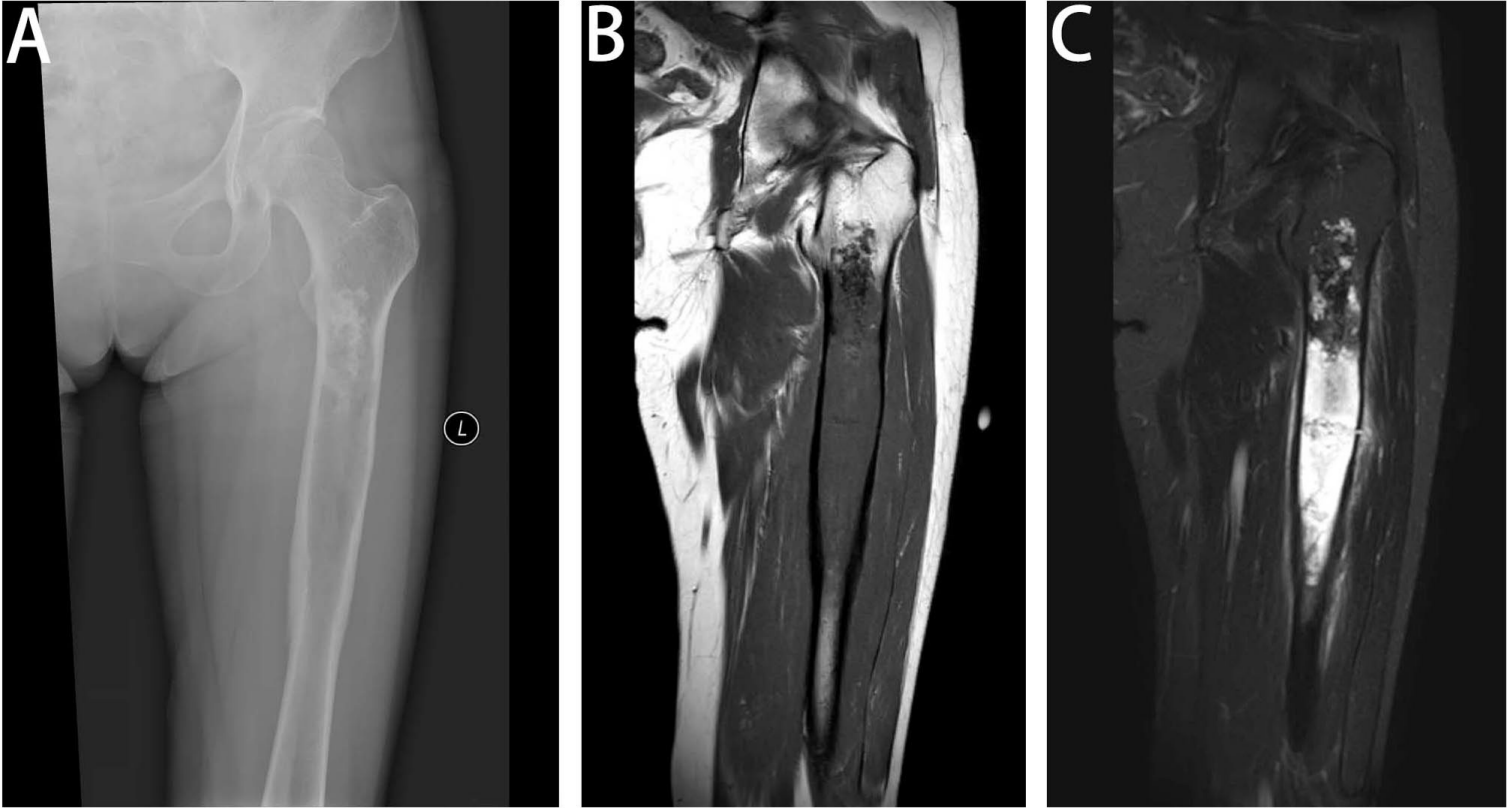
Radiologic features of DCCS. (A) Anteroposterior view of the left femur. Radiographs show a large lytic lesion of the femur, with cortical remodeling and a poorly defined margin of the distal part. The proximal part of the lesion has popcorn-like matrix calcifications. (B) Coronal T1-weighted MR image of the left femur. MR imaging shows a large destruction in the medullary cavity of the femur, with low signal on the T1-weighted image. (C) Coronal fat-suppressed T2-weighted MR image of the left femur. On fat-saturated T2-weighted images, the existence of the two components is recognized. The proximal third of the lesion has areas of low signal representing matrix mineralization and areas of high signal representing the high water content of the cartilage matrix, but the distal two-thirds has a heterogeneous predominantly high signal and peritumoral edema, showing bimorphism of the lesion.
